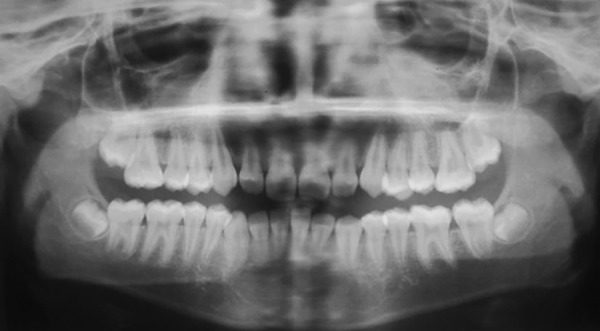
Postoperative radiograph with proper alignment of teeth
